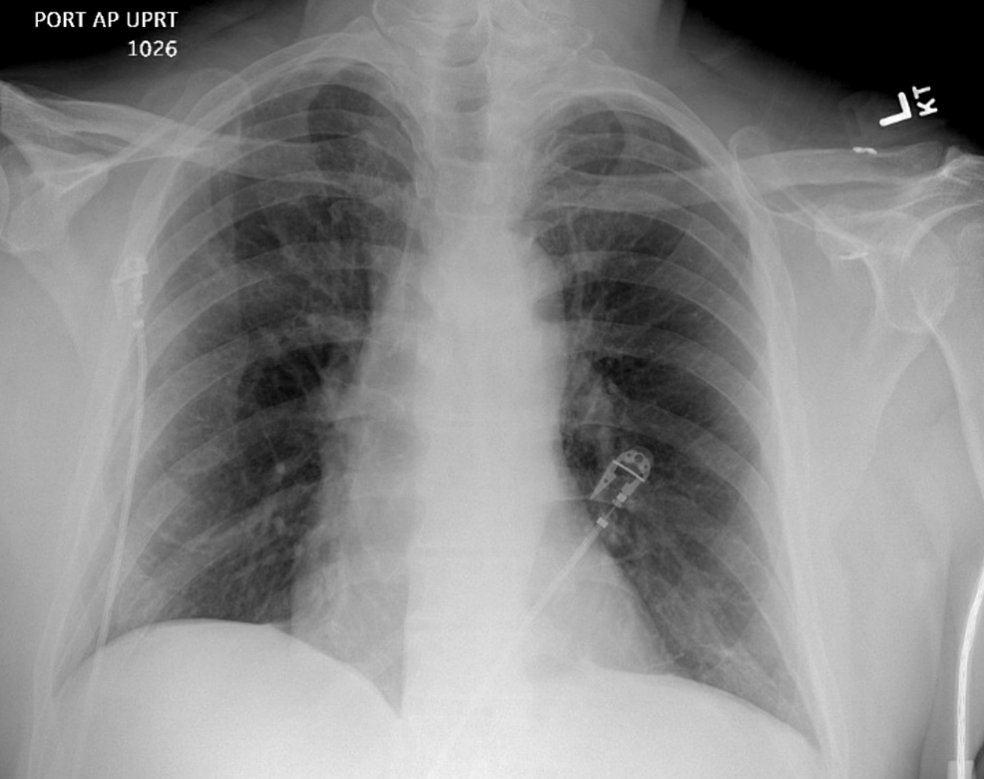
Chest x-ray after removal of pigtail catheter
